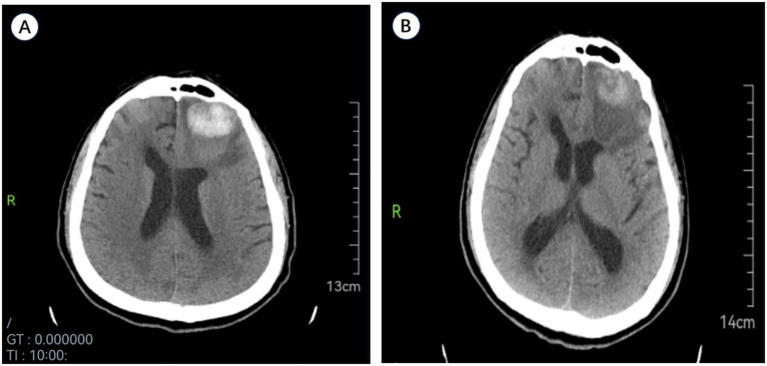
Follow-up cranial CT scans on (A) June 30, 2025, and (B) July 8, 2025, depicting a progressive reduction in the size of the left frontal lobe hematoma.
